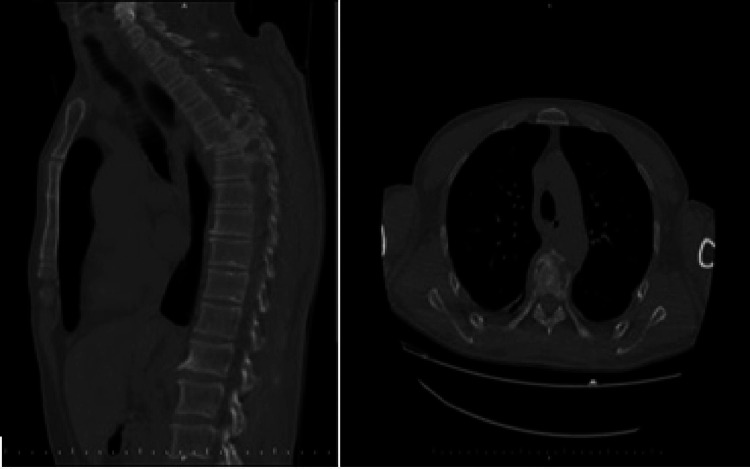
Preoperative CT view of the upper thoracic fracture.
